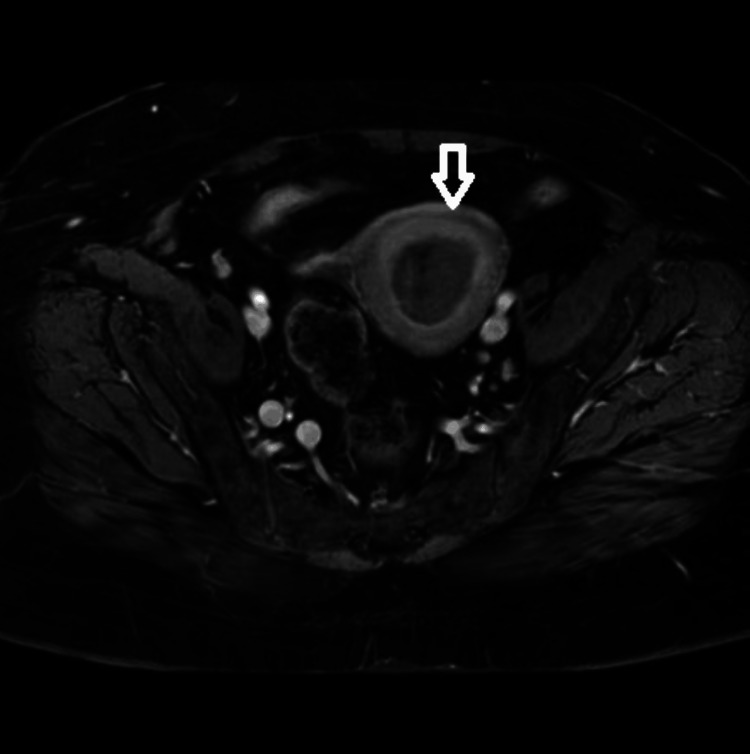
Axial T1-weighted contrast-enhanced MRI of the pelvis showing a lesion in the fundal wall of the uterus on the left side MRI: magnetic resonance imaging
